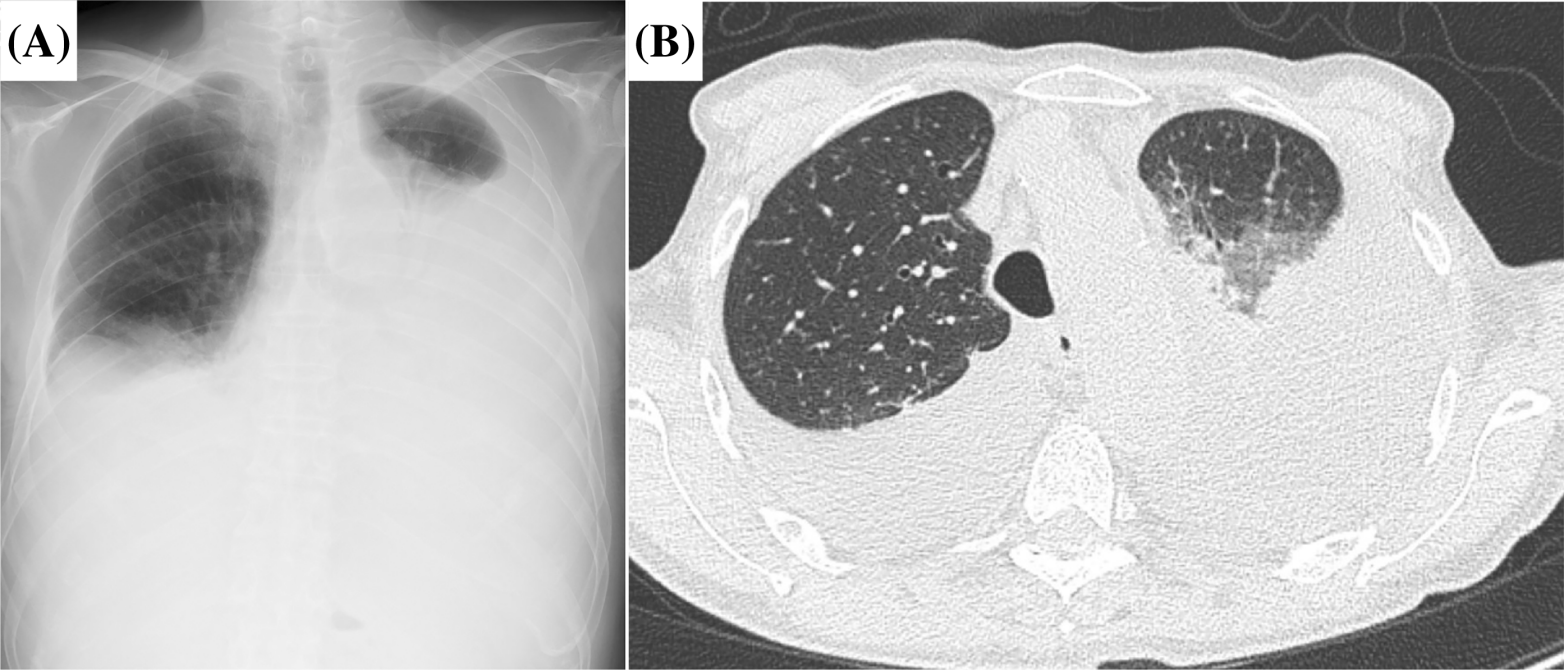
Chest x‐ray on admission showing bilateral moderate to large pleural effusion (A). Chest computed tomography showing collapsed lungs with significant bilateral intrathoracic fluid accumulation (B).
